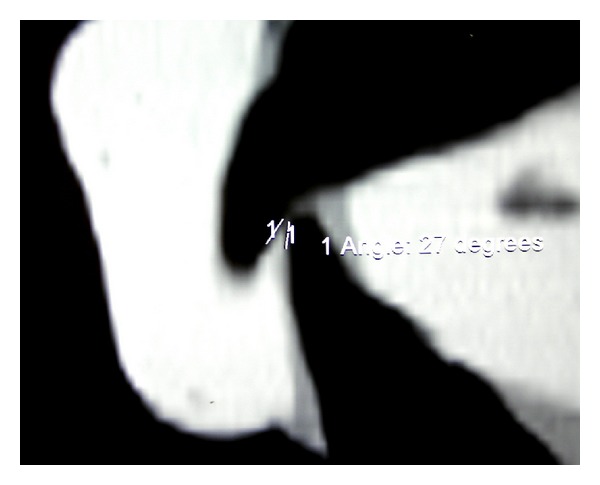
An individual IÔA calculated from CT image in the sagittal plane.
